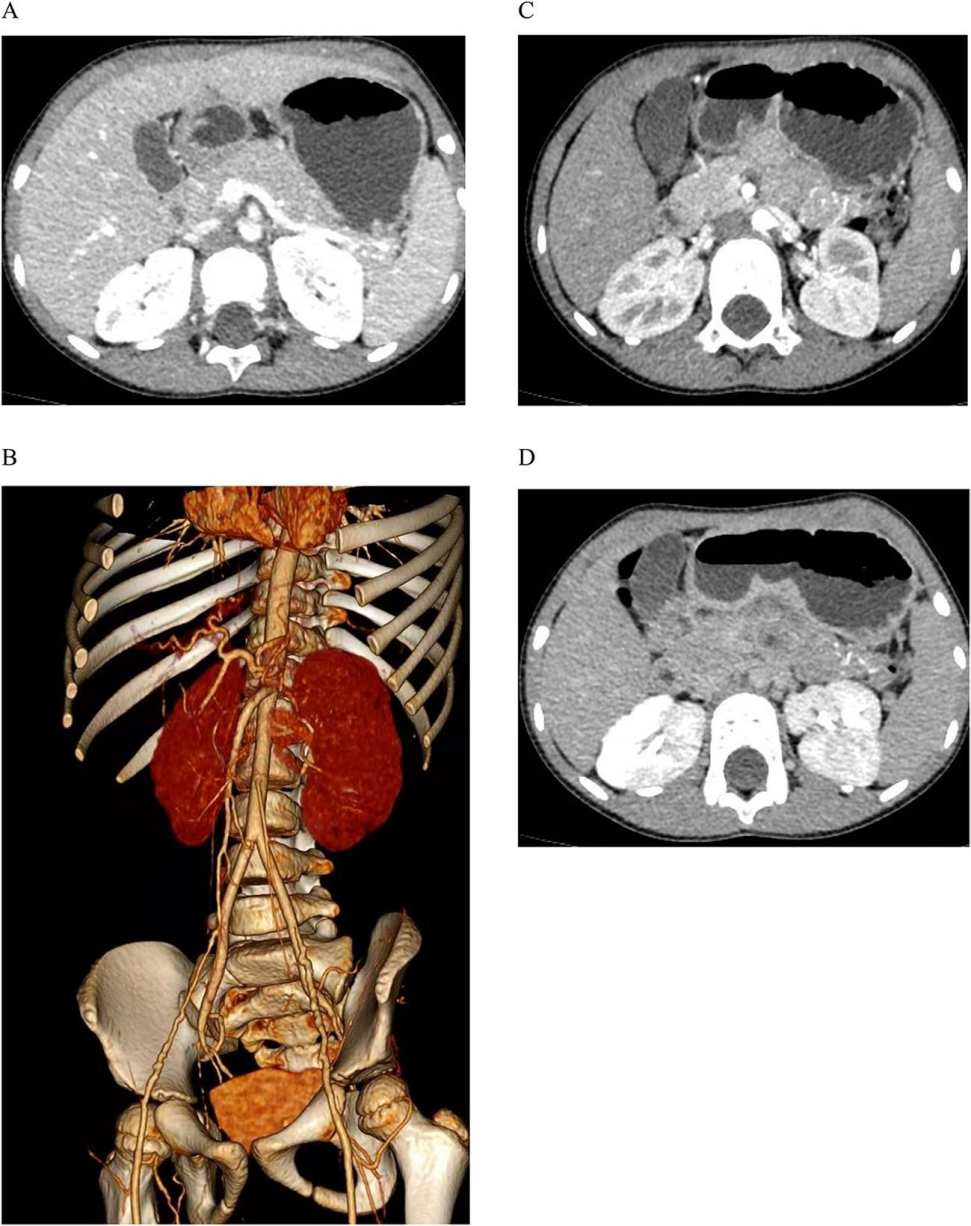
CTA of the abdominal pelvis. A Splenic artery imaging was not clear and splenic blood perfusion was reduced. B There were multiple tortuous and tiny vascular shadows in the tail of the pancreas. C No stenosis or malformation of the renal arteries was found. D Perfusion of the renal parenchyma is not uniform
